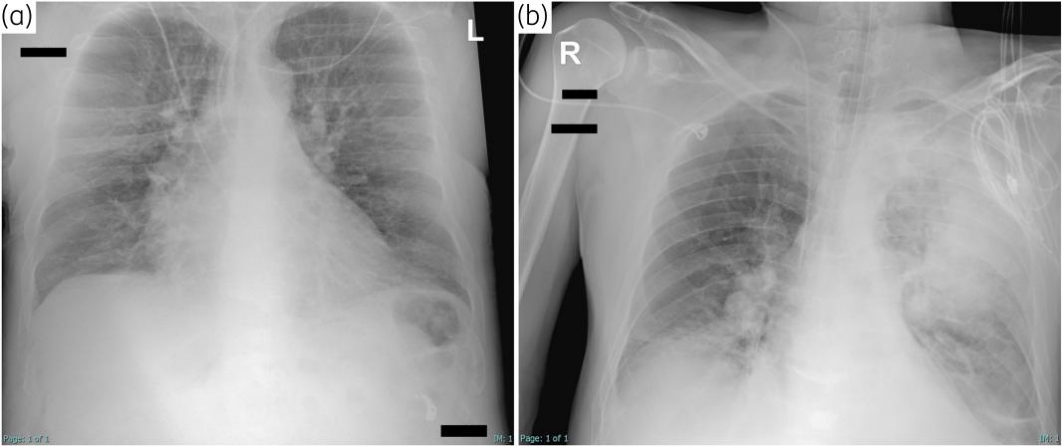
Chest X-ray performed at hospital admission (a) (no pathological findings) and 7 days later at the onset of respiratory failure and septic shock (b), which shows extensive lung consolidations in the lower right lobe and in the upper and lower left lobes.
